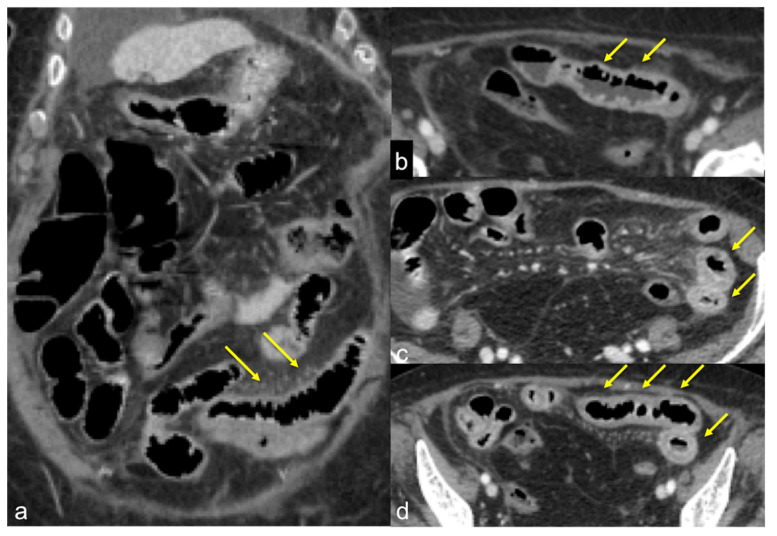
Reactivation of Crohn’s ileitis. Enhanced CTA scan in portal venous phase in coronal (a) and axial (b–d_b;c;d;d;e;f;d;e;f;a;b;c;a;b;c;d_) views. Note the ileal wall thickening with segmental irregularity and hyperaemia of the mucosa (a–d, arrows).
